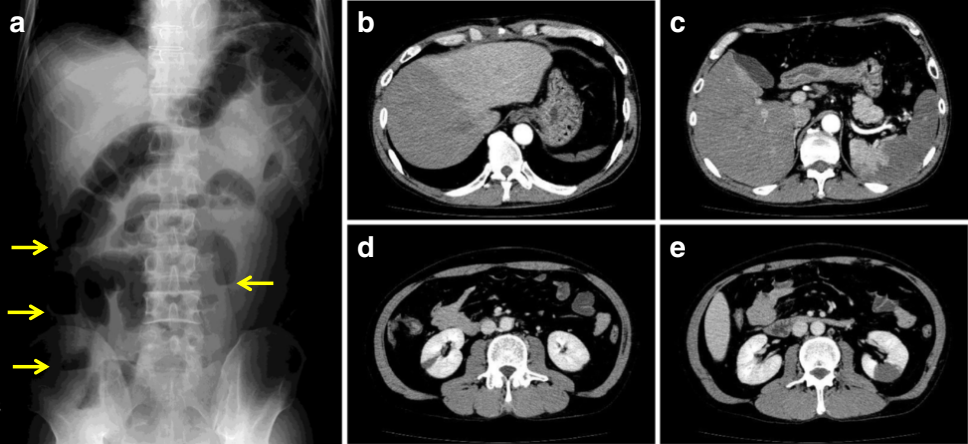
a. Dilated intestines and niveau (arrows), consistent with ileus, are present on this plain radiograph of the abdomen. b–e. 64-row, multi-detector, abdominal computed tomography showing hepatic b, splenic c and bilateral renal d & e infarctions
